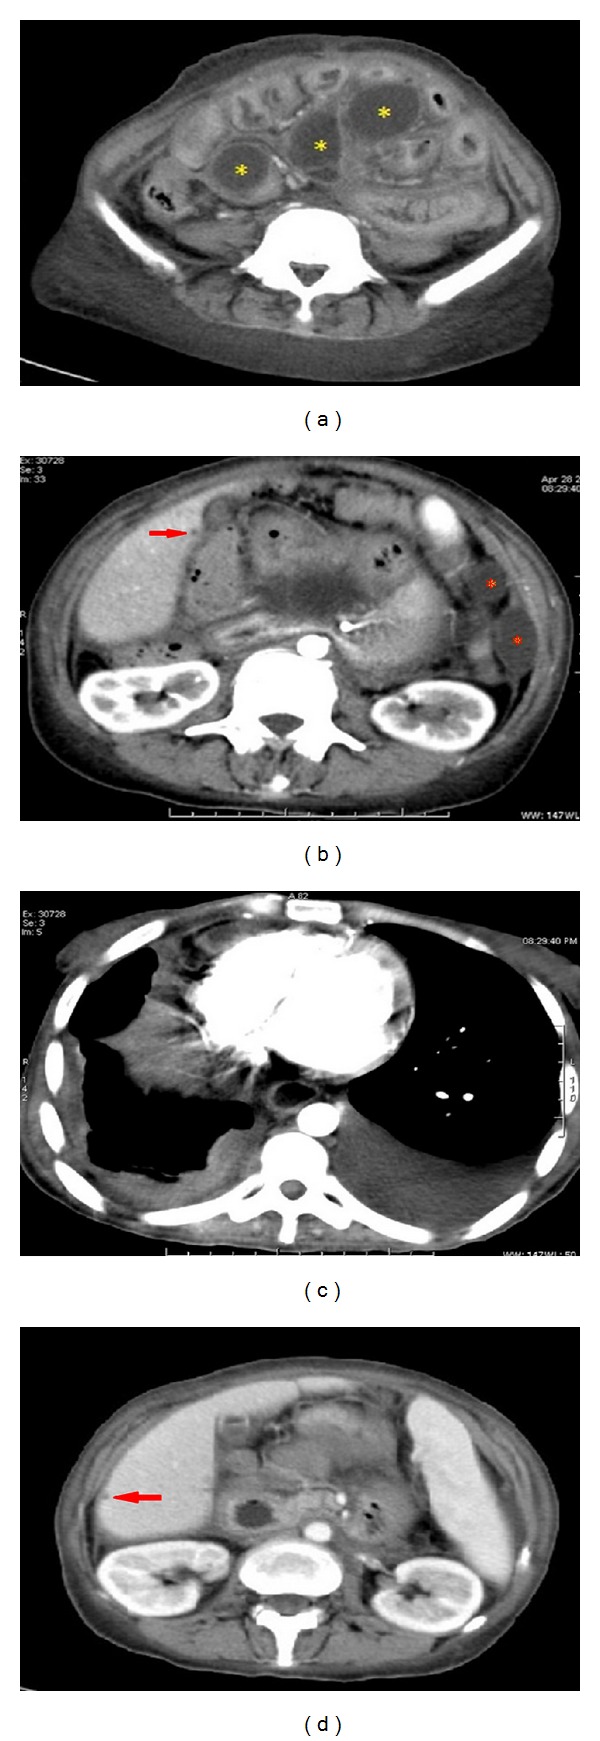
(a) Portal venous phase CT image. Multiple hypodense mesenteric thick walled cystic masses (gold stars) with diffuse intestinal wall thickening. (b) Arterial phase CT image with contrast. Subcentimetric hypodense hepatic focal lesion seen in segment V (red arrows) and two hypodense extraperitoneal cystic lesions seen in left lumbar region (red stars). (c) Bilateral pleural effusions, left side greater than the right. (d) Similar hepatic focal lesion as seen in (b) (red arrow).
